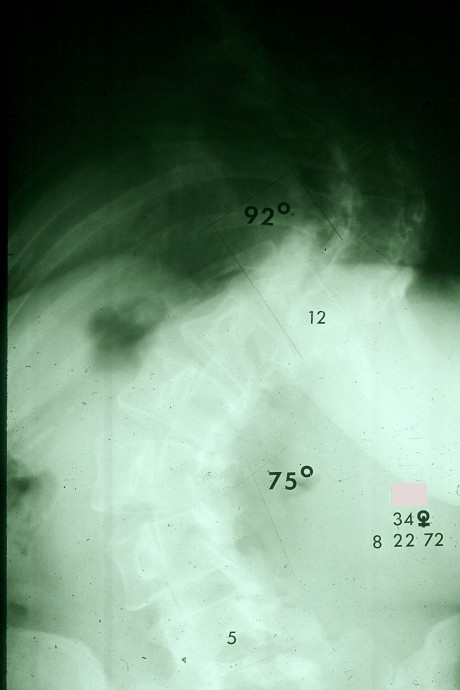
Posterior-anterior radiograph of a women with  double major scoliosis taken at age 34 years. (Reprinted with permission from Asher M, Burton DC: Natürlicher verlauf und langzeitauswirkungen der idiopathischen adoleszentenskoliose. In *Wirbel Säulen Deformitäten: Konservatives Management*. Edited by Weiss HR. München: Pflaum; 2003:97-107.)
